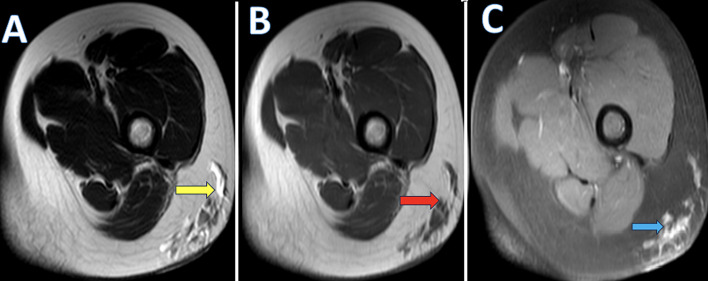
axial magnetic resonance imaging images of the left thigh demonstrating multiple well-defined cystic lesions in the subcutaneous plane of the posterolateral aspect of the thigh (arrows); A) T2-weighted image showing hyperintense cystic lesions (yellow arrow) in the subcutaneous tissue; B) T1-weighted image demonstrating hypointense signal intensity of the same lesions (red arrow); C) post-contrast T1 fat-suppressed image showing minimal thin peripheral enhancement of the cystic lesions (blue arrow)
